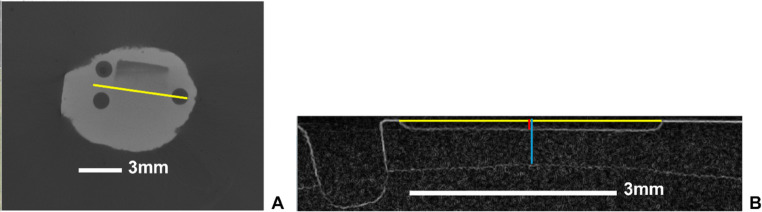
Reference line bisecting a lesion. To analyze the µ-CT images, individual DICOM files were imported into ImageJ, generating a volumetric reconstruction of the sample from a facial perspective. The examiner then scrolled through the image until the three reference points were visible and drew a line (yellow) bisecting the one point incisal to the lesion and the two points gingival to the lesion (a). A function called *kymograph* builder was then utilized to view the image from the sagittal position. A line (yellow) was drawn across the top of the lesion and another line (red) bisecting the first was drawn to the depth of the lesion and measured. A vertical line (shown in blue) was also drawn to measure the depth of the enamel in the same location (b)
